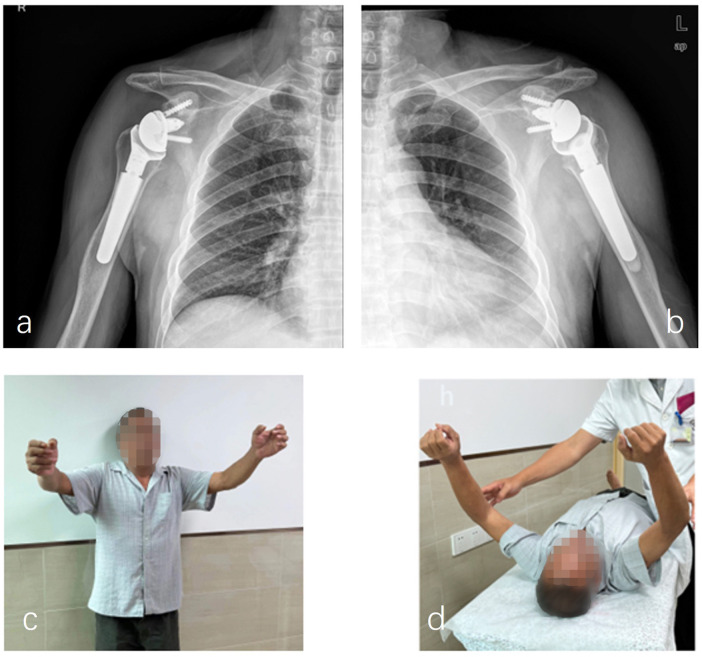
(a,b) A postoperative assessment of shoulder function in the clinic at 3 months. (c,d) Postoperative anteroposterior (AP) radiographs of both shoulders demonstrating prosthesis stability.
